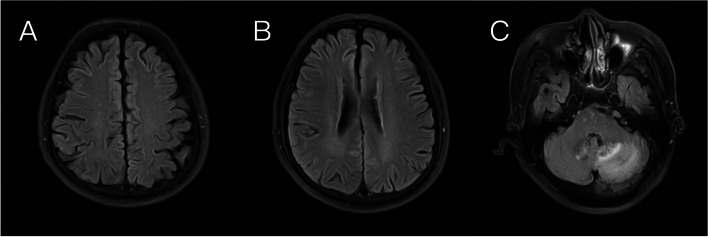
The brain MRI after tislelizumab therapy in August 2021. A, B Compared to that of April 2021, the lesions in the bilateral frontal lobe vanished. C The lesions in the right temporal lobe were significantly reduced
